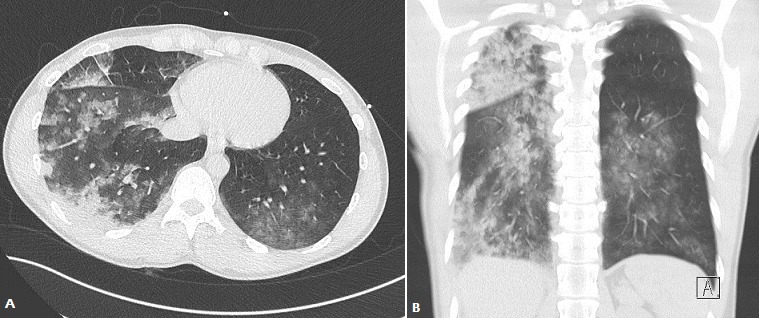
Axial (A) and coronal (B) non-contrast-enhanced–CT scan shows multiples images of alveolar opacity in the right lung and lesser in the contralateral lung
